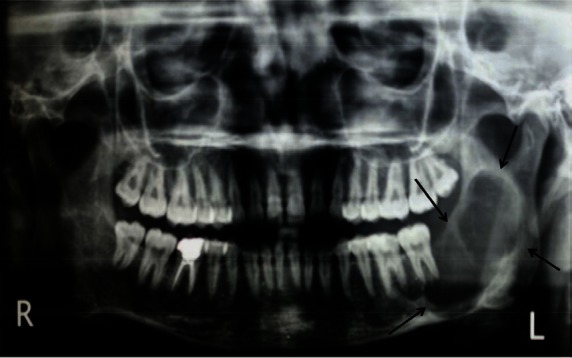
Panoramic radiograph showing multilocular radiolucent lesion in ascending ramus
